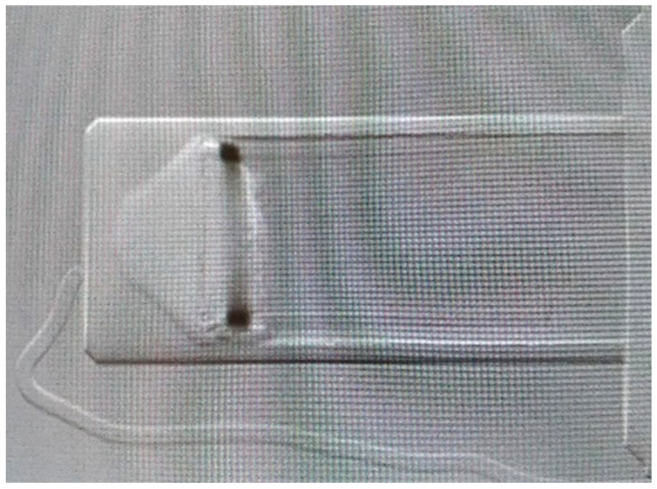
Neutron radiograph of an in situ hydrogen diffusion experiment with ZrH_2_ powder in a Zry-4 cladding tube at the BOA beamline at the PSI.
